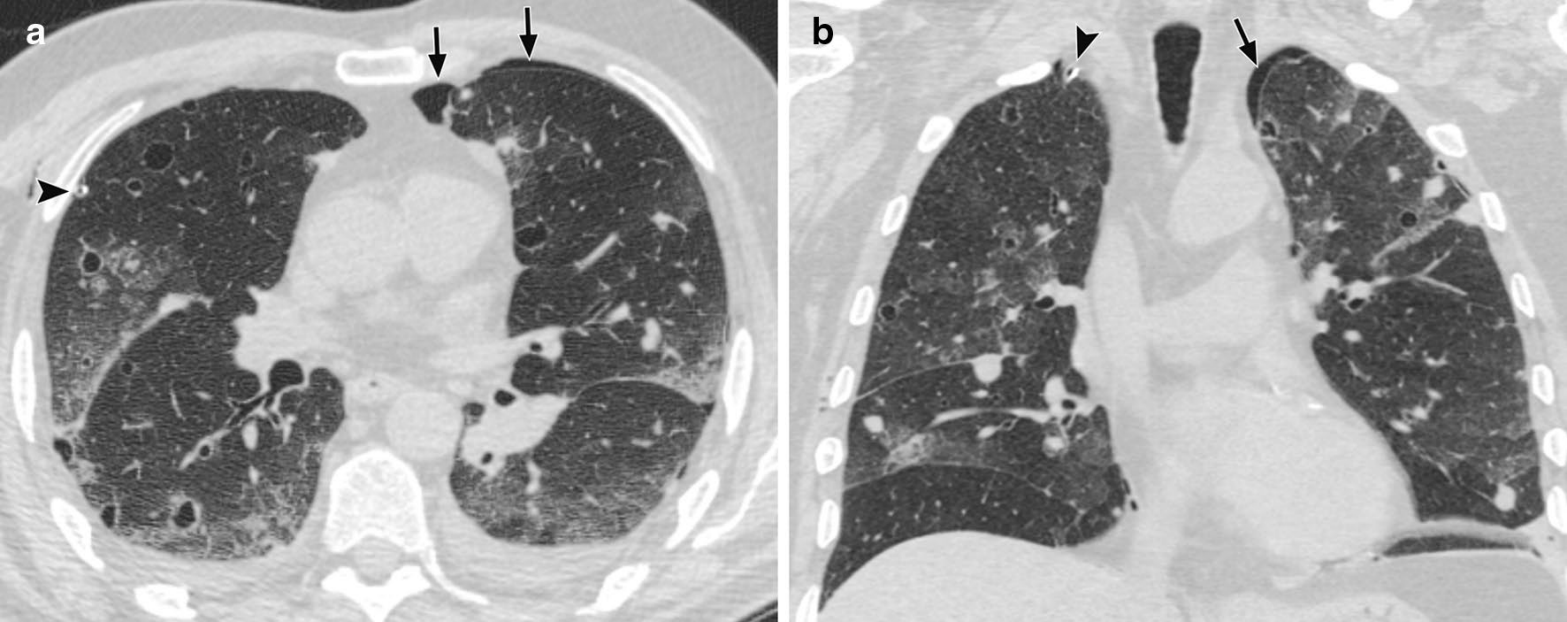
65-year-old with radiation-associated angiosarcoma of the parotid gland and pulmonary metastases. a Axial and (b) coronal CT (lung windows) images show multiple nodule and cysts in the lungs, some of which are surrounded with ground-glass changes. There is a small left-sided apical pneumothorax (arrows). There is also a chest drain within the right pleural space due to a previous right pneumothorax (arrowhead)
